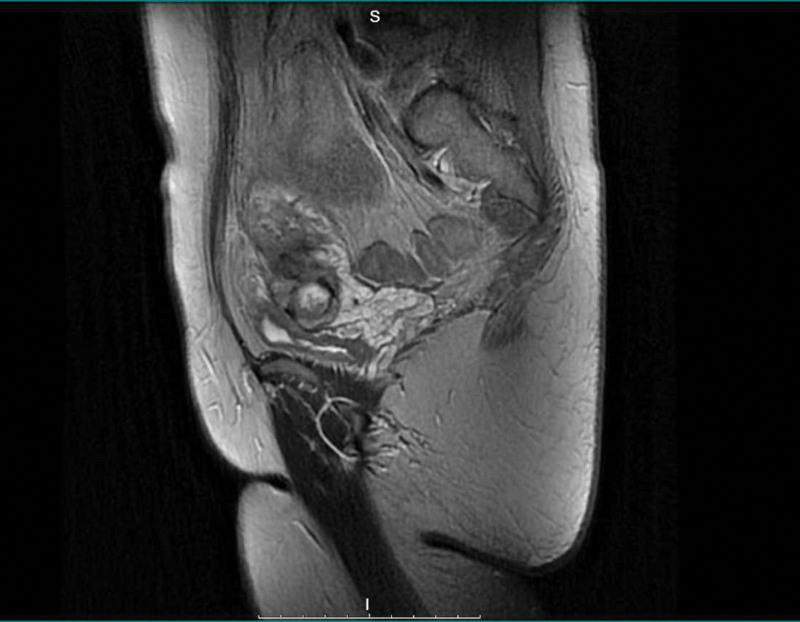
First postoperative MRI image.
